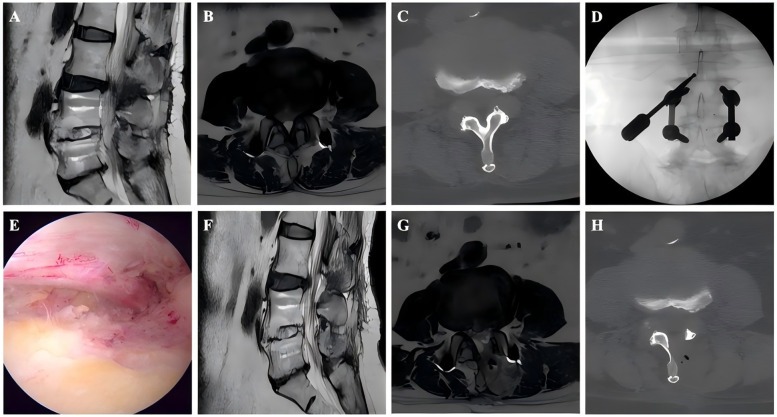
Images from an ASD patient treated with UBE. A–B: Preoperative MRI showed that the L4–L5 had undergone PLIF surgery previously and L3–L4 LSS. C: Preoperative CT. D: The position of the grinding drill was shown in the fluoroscopic view. E: The nerve was completely decompressed. F–G: Postoperative MRI confirmed spinal canal decompression. H: Postoperative CT showed a lamina defect after partial laminotomy.
